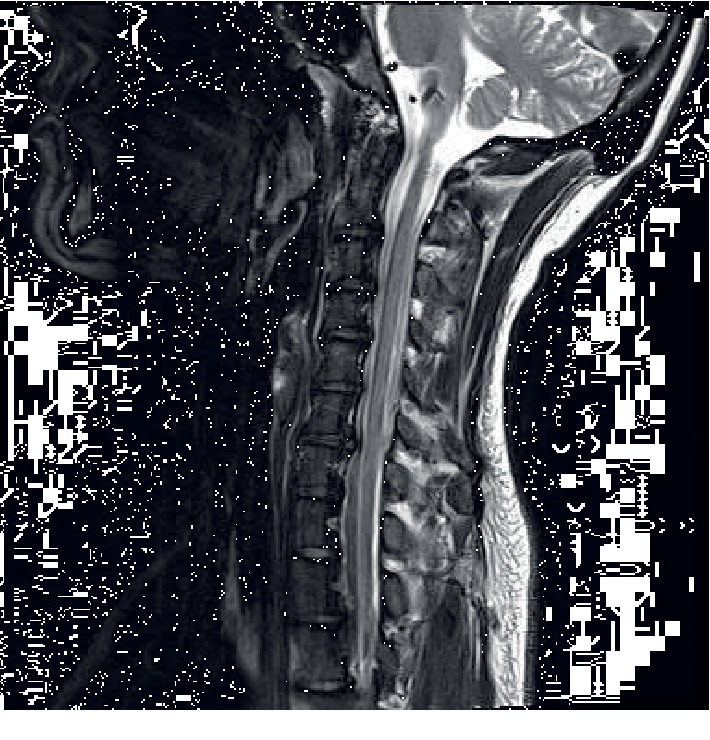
MRI of cervical spine. MRI of the cervical spine revealed findings suggestive of mild diffuse spinal cord atrophy.
